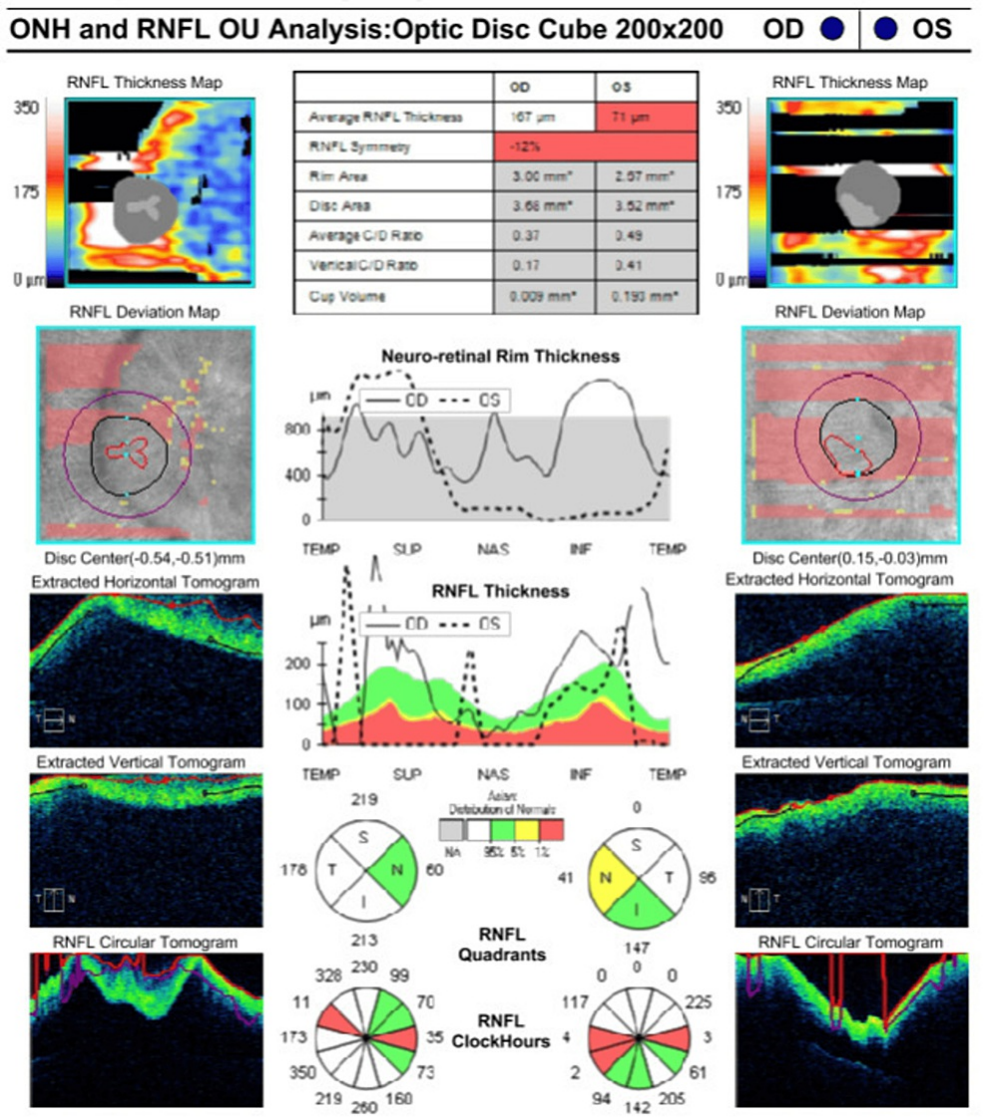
Ocular computed tomography image of optic nerve head and retinal nerve fibre layers showed swollen and abnormal optic disc.
